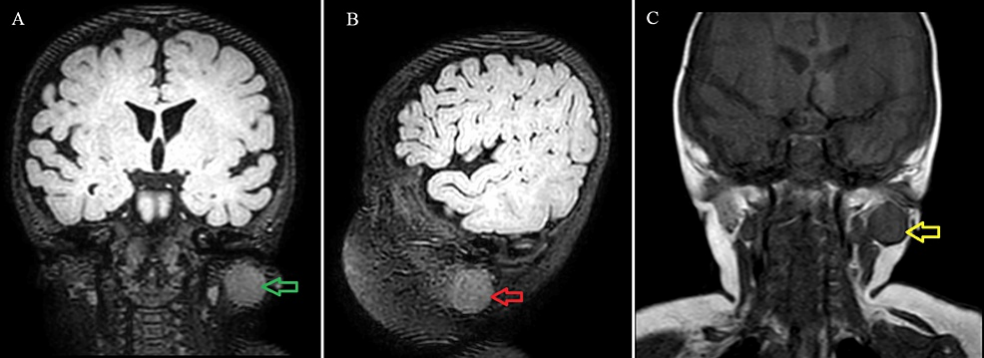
(A) Coronal MRI cut showing a hyper-intense well-circumscribed mass on T1 (green arrow). (B) Sagittal MRI cut showing a hyper-intense well-circumscribed mass on T1 (red arrow). (C) Coronal MRI cut showing a hyper-intense well-circumscribed mass on T2 (yellow arrow). MRI: magnetic resonance imaging
